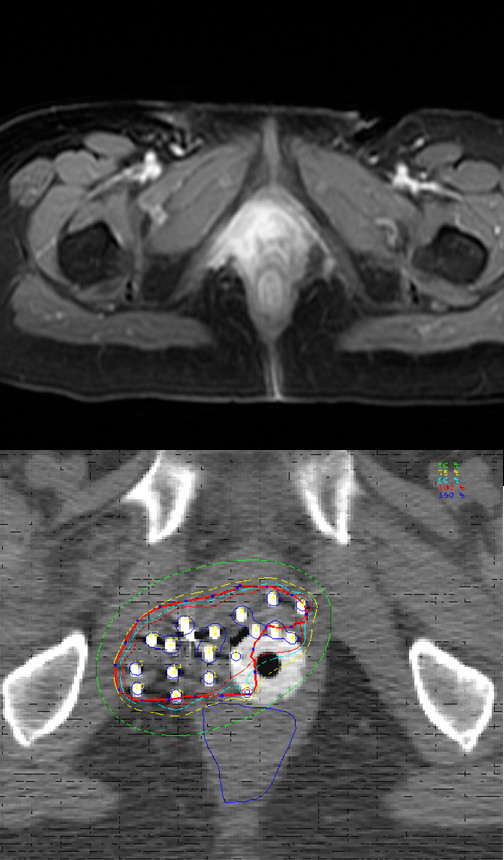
Pre-RT MRI image (T1 with contrast) showing large vaginal cancer with extension to pelvic side wall and peri-urethral region. Interstitial implantation showing coverage of clinical tumor volume (red) with isodose lines (100%-red, 90%-blue, 75%-yellow, and 50% – green).
